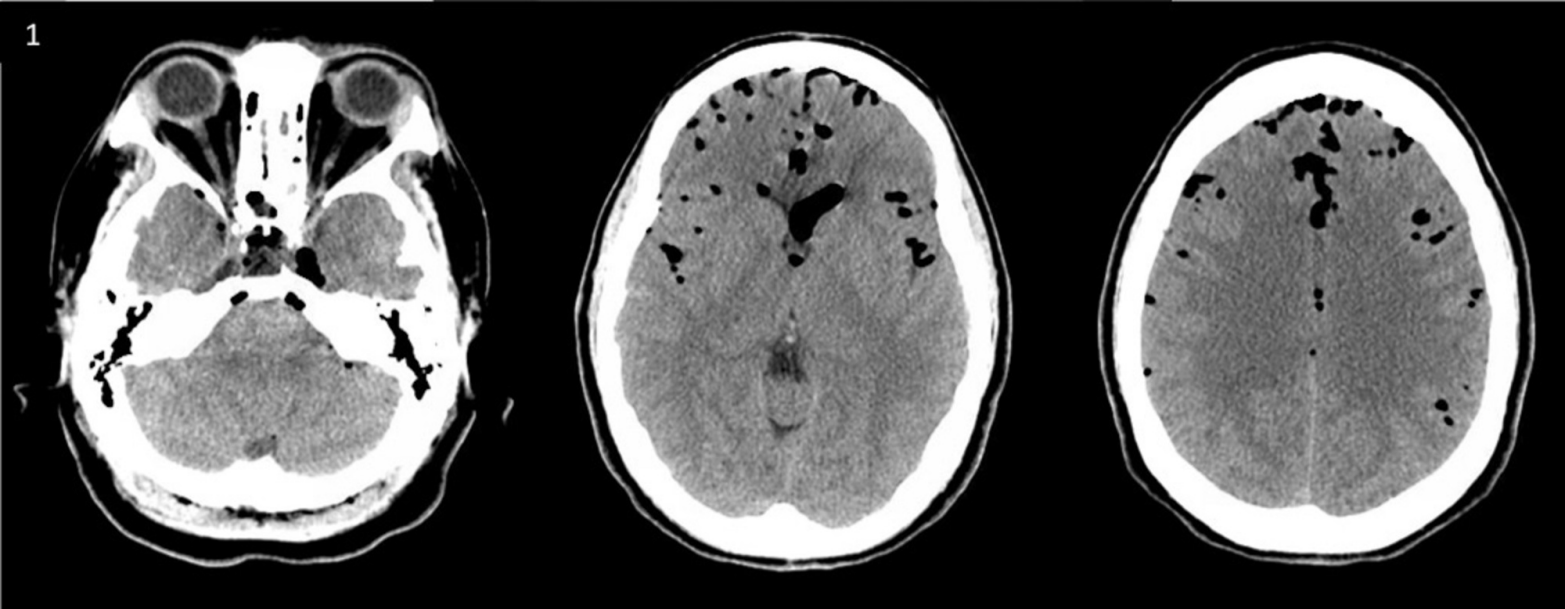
The first picture demonstrated an evidence of pneumocephalus (PNC). Axial computed tomography images of the brain on the first day in the emergency room showed diffuse PNC most pronounced in the frontal region.
